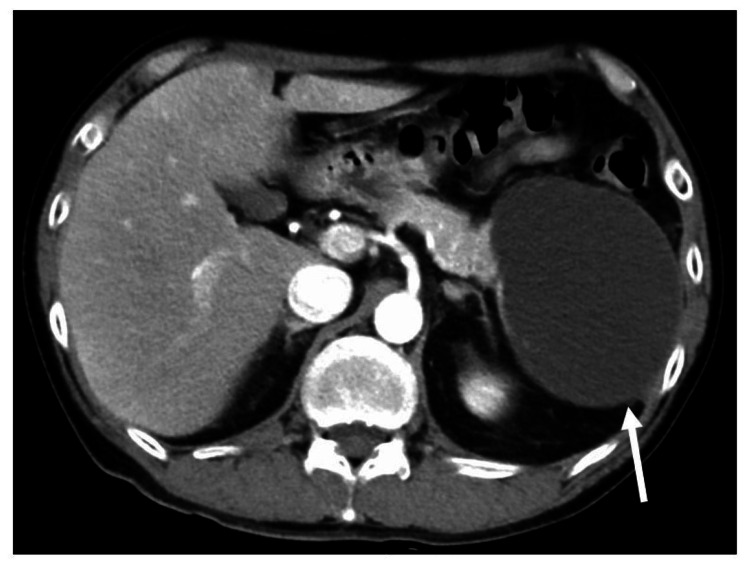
Computed tomography performed 10 months after the initial hospital visit. Computed tomography revealed the pancreatic cyst had grown to 10 cm in diameter (arrow).
